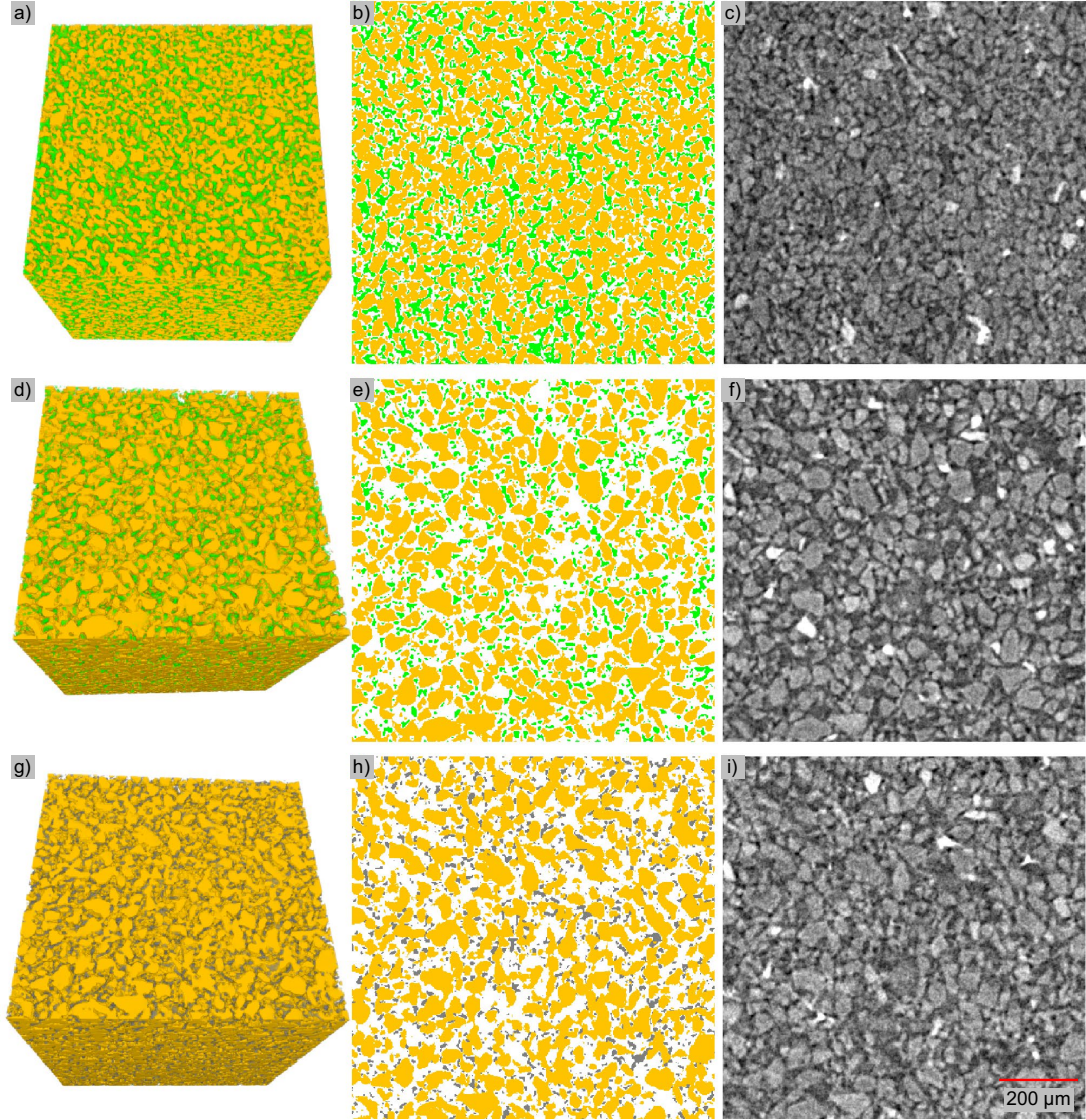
Visualization of segmented CT images. Figures (a–f) show hydrate-bearing pressure core (10 ºC, 24.1 MPa, within hydrate stability zone), (a–c) have a higher saturation than (d–f). Panels (g), (h), and (i) (24 ºC, 11 MPa, outside hydrate stability zone) are roughly the same area as (d), (e), and (f) after hydrate dissociation. Segmented results are shown in 3D (a, d, and g) and 2D (b, e, and h). Images in (b), (e), and (h) correspond to the top slice in (a), (d), and (g). Sediment particles, hydrate particles and gas bubbles are in yellow, green and gray respectively, and pore fluid is made transparent. Raw images of (b), (e), and (h) are shown in (c), (f), and (i). Sediment particles, NaI doped pore fluid, gas hydrate, and free gas are with decreasing grayscale values in (c), (f), and (i).
